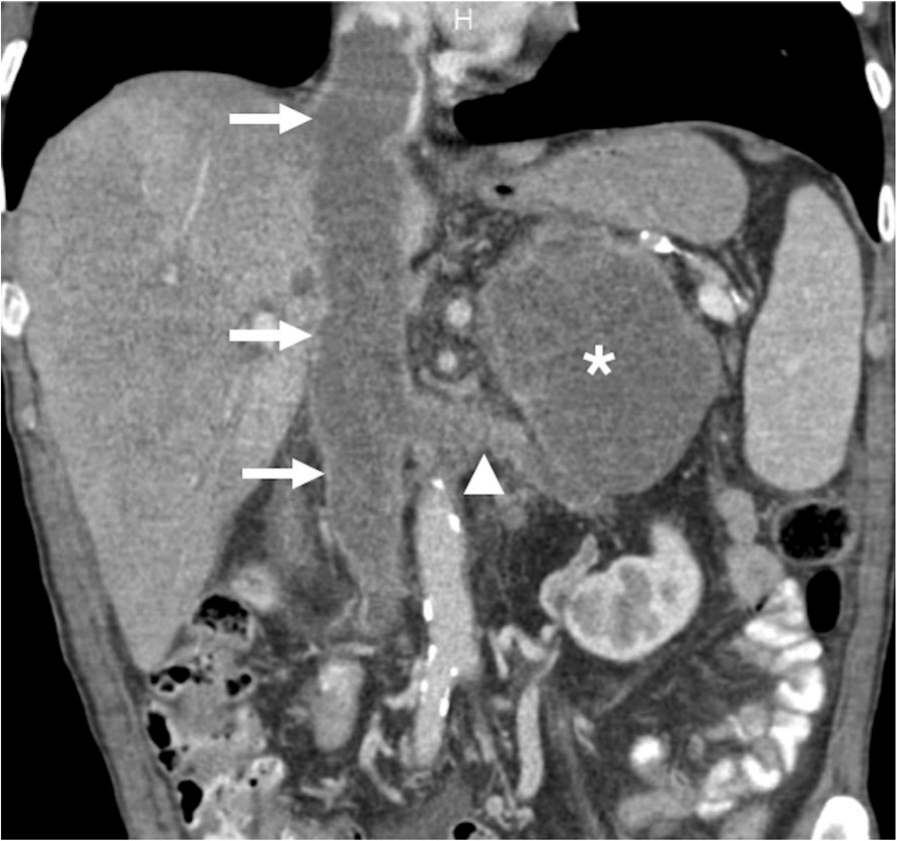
A 60-year-old male with no past medical history presented with left flank pain and weight loss. Coronally reformatted postcontrast CT image shows a left adrenal mass (asterisk) invading the left renal vein (arrowhead) and IVC (arrows). Surgical resection revealed adrenocortical carcinoma with tumor thrombus extending into the left renal vein and IVC
